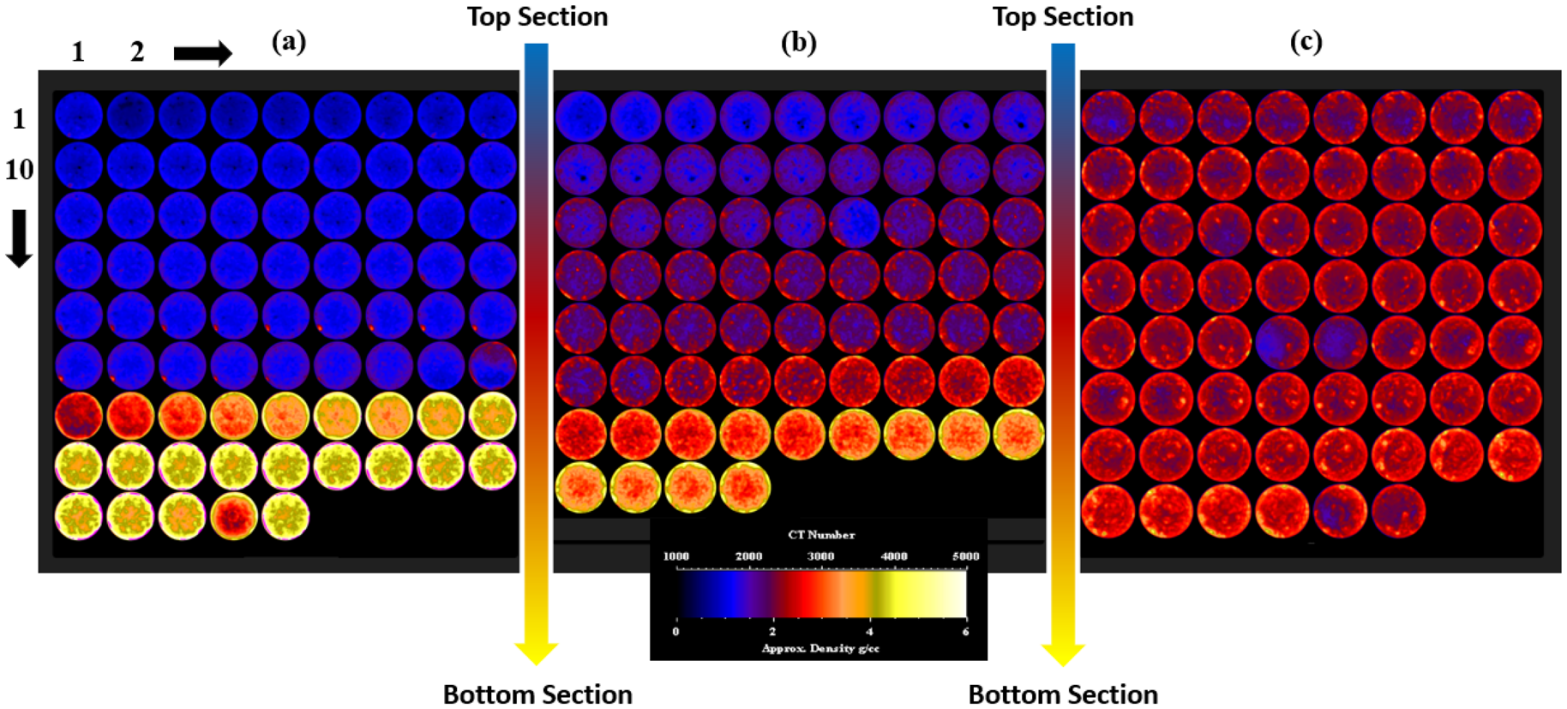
CT-scan results for different perlite concentrations [0% (a), 1.5% (b) and 3% (c)].
